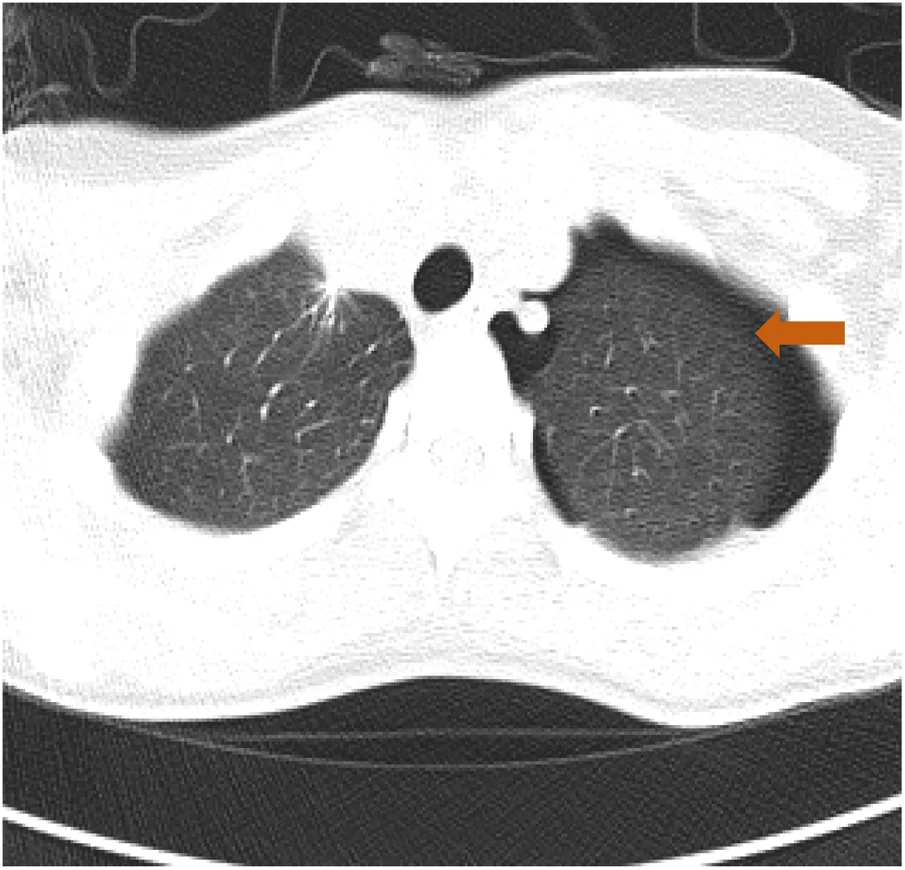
Preoperative chest CT of the second surgery. Left pneumothorax with 50% lung compression.
